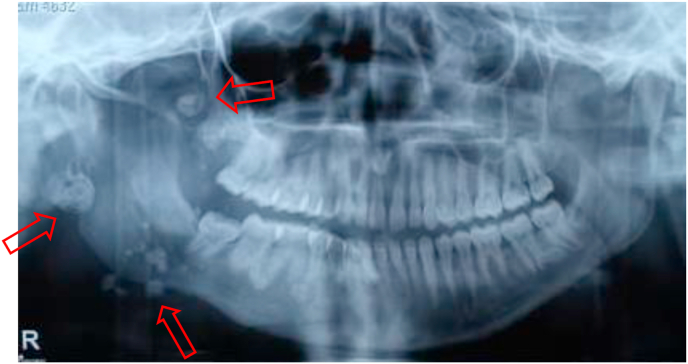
X-ray showing pheboliths in the right mandible with caries in the 48.
